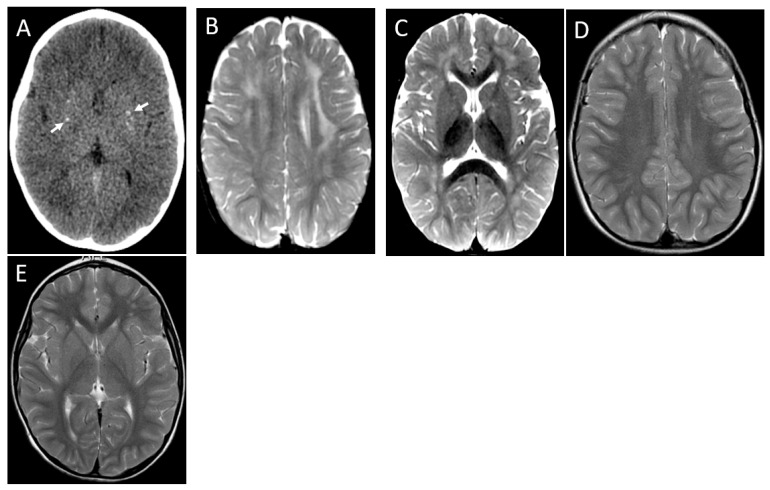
Patient 4. Axial CT image (A) demonstrates small foci of bilateral basal ganglia calcification (arrows). First MRI images at 17 months, axial T2 (B,C) showing bilateral frontoparietal asymmetric areas of signal hyperintensity. The second MRI at 3 years shows near complete resolution of leukopathy (D,E).
